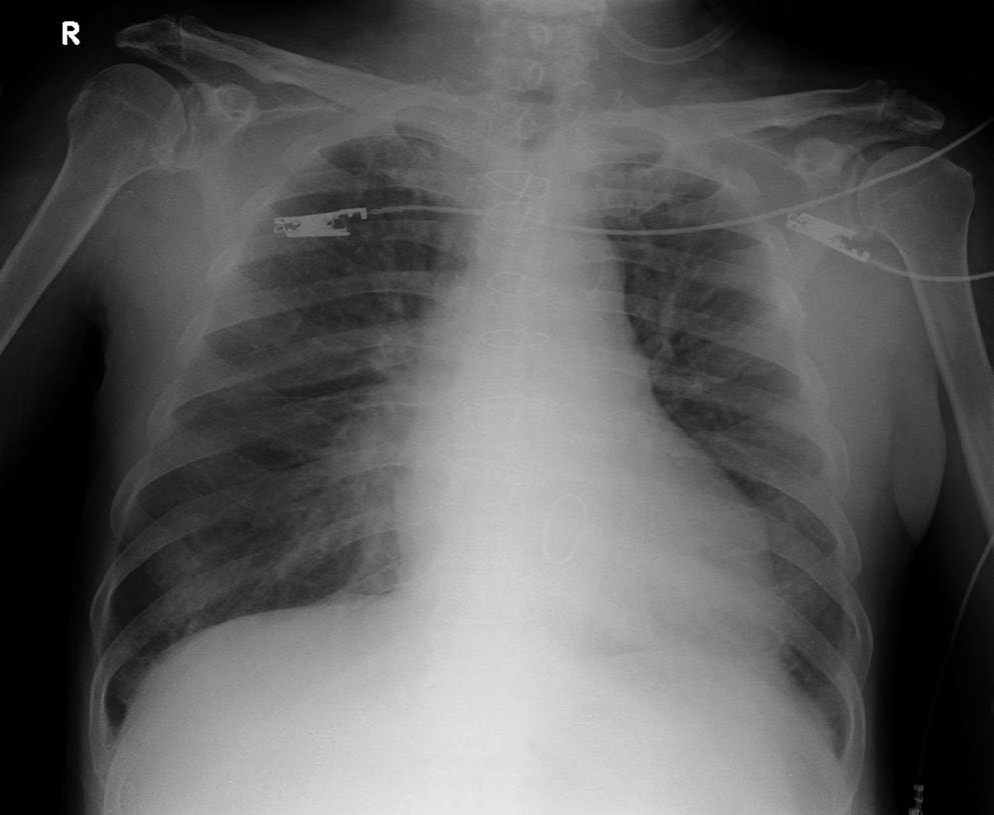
Admission chest radiography. Anteroposterior image reveals mild heart enlargement and slightly increased pulmonary vascular markings
